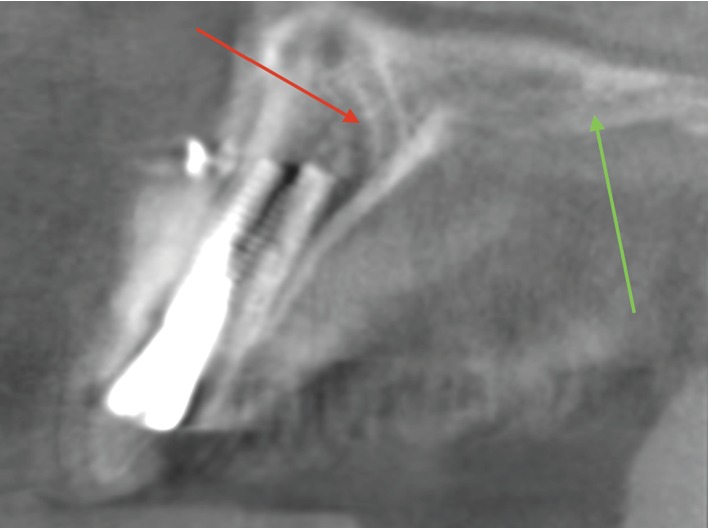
Postoperative CBCT scan, sagittal view in the left maxillary incisor region. The dental implant in situ. The diagnosis is difficult due to artefacts caused by the titanium implant; however, the major palatal branch of the CS could be seen (red arrow). Also, the bony contours of the left greater palatal artery and nerve are visualized (green arrow).
